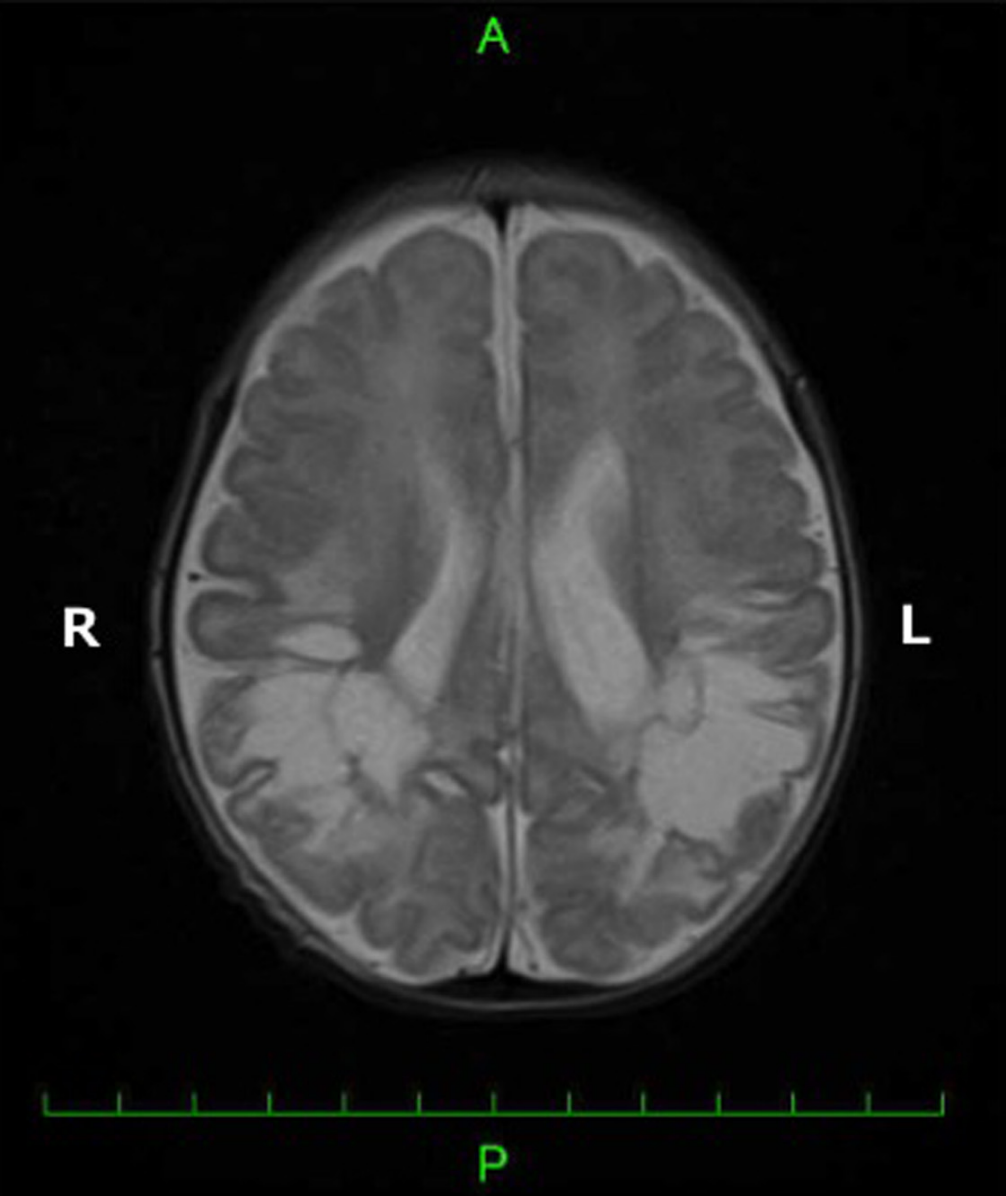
Large periventricular leukomalacia diagnosed on the basis of magnetic resonance imaging findings (T2‐weighted imaging sequences in the transverse plane).
